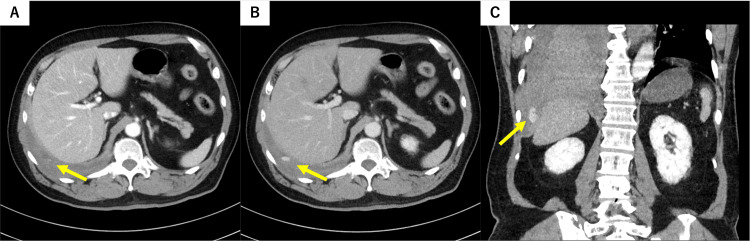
Contrast-enhanced CT identifying a pseudoaneurysm near the diaphragm. (A) Arterial-phase axial image showing a focal contrast blush along the right diaphragmatic surface (arrow). (B) Portal venous-phase axial image with persistent enhancement (arrow). (C) Coronal reformatted image demonstrating a saccular lesion abutting the parietal pleura near the right 10th rib (arrow), consistent with a pseudoaneurysm.
